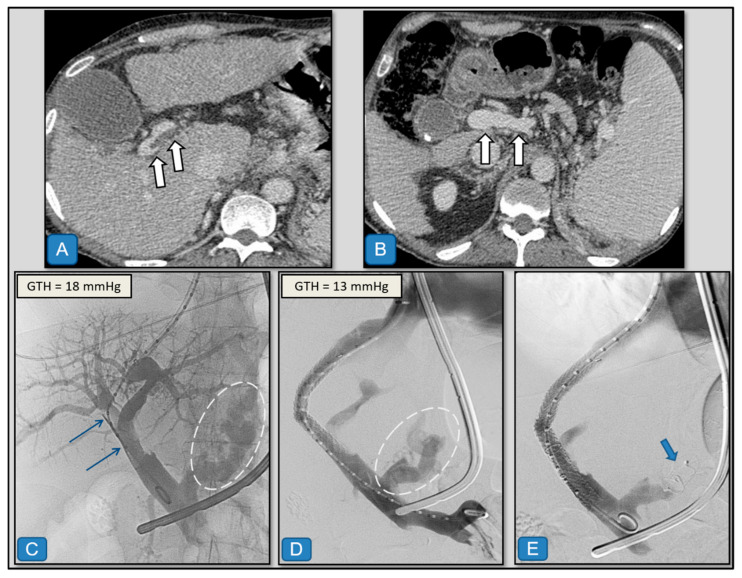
Classic transjugular approach in a 48-year-old man with alcoholic and hepatitis C virus infection cirrhosis with upper gastrointestinal bleeding due to gastroesophageal varices. (A,B) Contrast-enhanced computed tomography, axial images in portal venous phase. Cirrhotic liver with a partial acute thrombus in the right portal vein (arrows in (A)), with patent porta hepatis and splenoportal axis (arrows in (B)). (C) Using a standard ultrasound-guided access through the right internal jugular vein, puncture was performed into the partially thrombosed right portal branch. Direct portography demonstrates an acute partial thrombosis of the main portal vein that extends to the right portal branch (blue arrows) and opacification of gastroesophageal varices (dashed ovals). The pre-TIPS transhepatic gradient (GTH) was 18 mmHg. (D) A 0.035″ hydrophilic guidewire and catheter were advanced through the thrombus, and a TIPS was placed. A 10 mm uncovered stent was deployed, overlapping toward the origin of the superior mesenteric vein, covering the partially thrombosed segment. Both stents were sequentially dilated with conventional 8 and 10 mm balloons, achieving a final post-TIPS GTH of 13 mmHg. Finally, embolization of the left gastric vein was performed with a 14 mm Amplatzer plug (AGA Medical Corporation, Golden Valley, MN, USA) (blue arrow in (E)).
